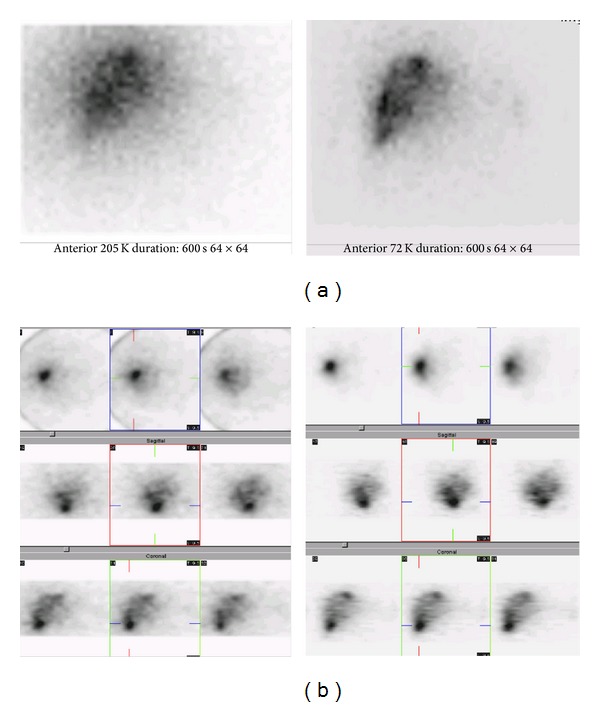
A patient with metastatic colon cancer treated with 333 MBq ^32^P particles. (a) Bremsstrahlung ³²P images in planar study with LEHR and MEGP collimators. (b) Bremsstrahlung ³²P images in SPECT study with LEHR and MEGP collimators.
